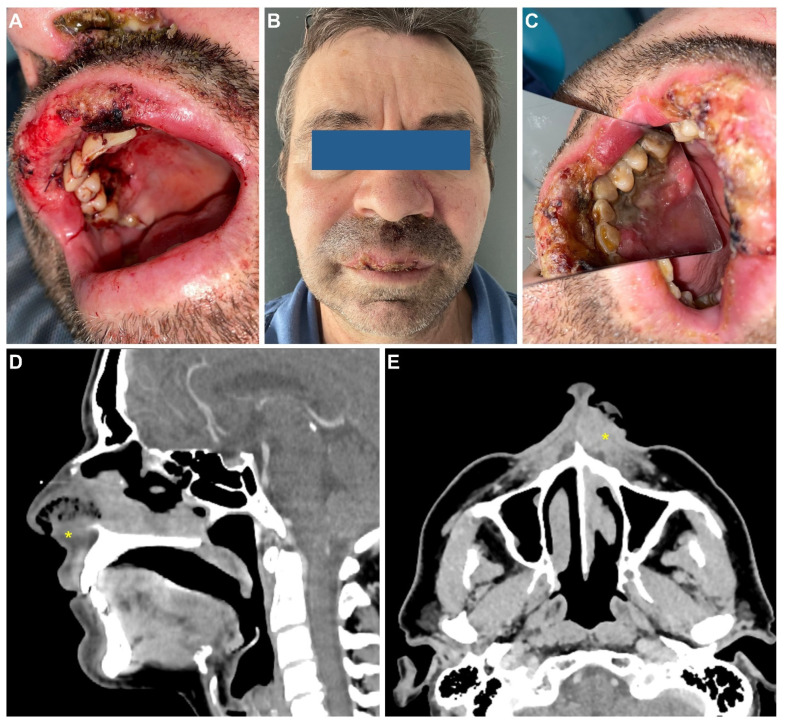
Clinical and imaging aspects at presentation. Intraoral aspect at the presentation to the emergency room, after superior labial artery ligature (A); clinical appearance after 2 days (B,C); contrast-enhanced CT scan of the neck in the sagittal (D) and axial (E) planes. Heterogeneous infiltrative mass (*) of the upper lip, left inferior nasal vestibule, and left inferior turbinate.
